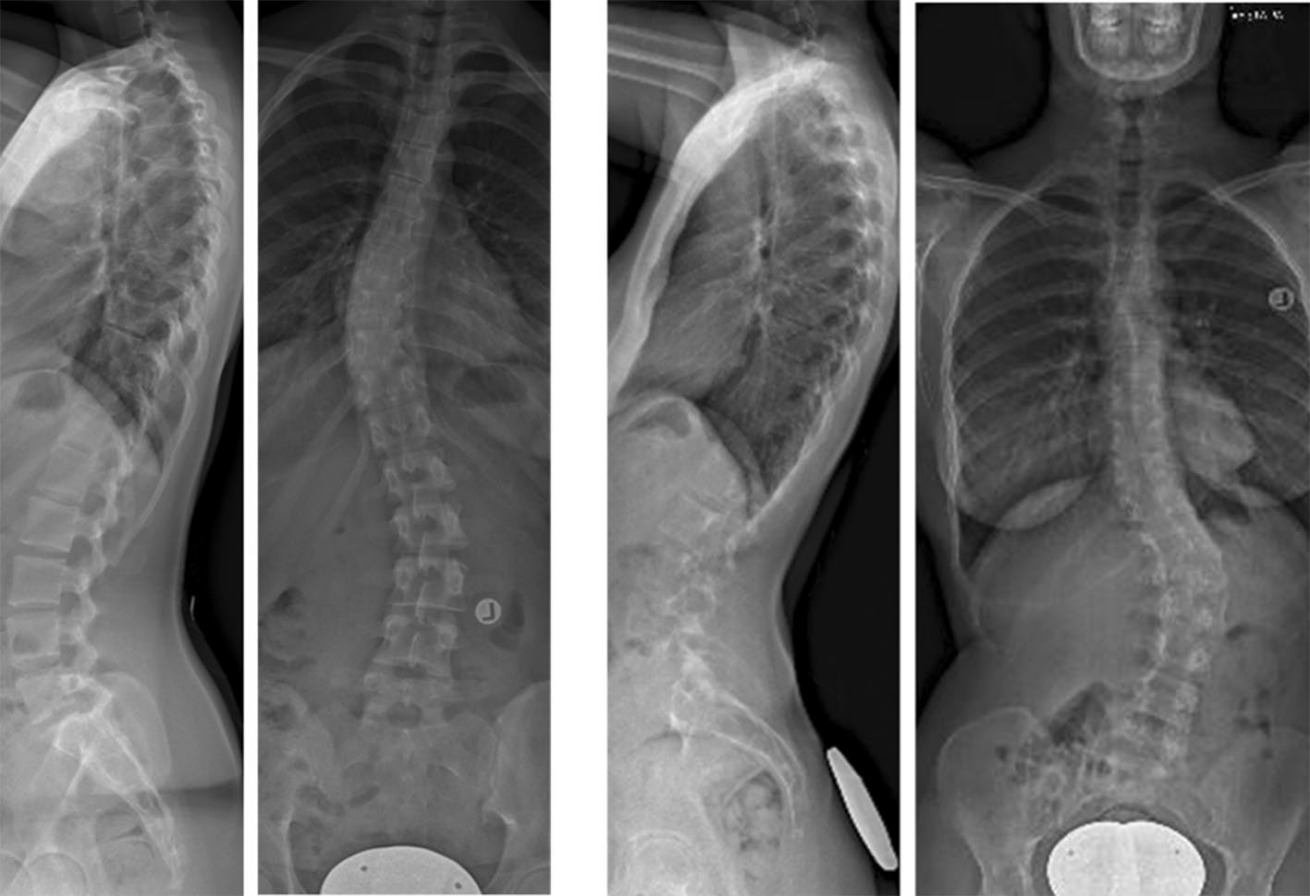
PA and lateral radiographs of a monozygotic twin pair. Radiographs were taken less than 3 months apart. This monozygotic twin pair was characterized by two different coronal curvatures (thoracic vs. thoracolumbar) also had two different sagittal profiles [small versus high pelvic incidence, Abelin-Genevois curve type 2a (hypokyphosis) versus 1 (normokyphosis)]
